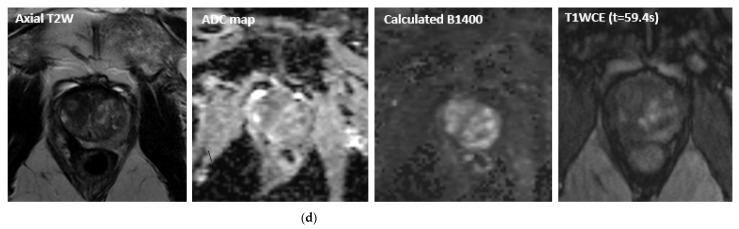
A 67-year-old patient with a PSA level of 4.6 ng/mL and Gleason 3 + 4 = 7 cancer in the right peripheral zone (PI-RADS 4). The lesion size is 7 mm in diameter. (a) Pre-treatment multiparametric MR images: Axial T2W image, ADC map, high *b* value and contrast-enhanced image, respectively. (b) Axial image of MR temperature mapping acquired during focal laser ablation (FLA). (c) Axial dynamic contrast-enhanced MR image acquired immediately after FLA demonstrating the non-enhancing ablation zone. (d) Post-treatment multiparametric MR images at one year follow-up (PSA level of 2.2 ng/mL): Axial T2W image, ADC map, high *b* value and contrast-enhanced image, respectively. There is no evidence of residual disease or recurrence.
